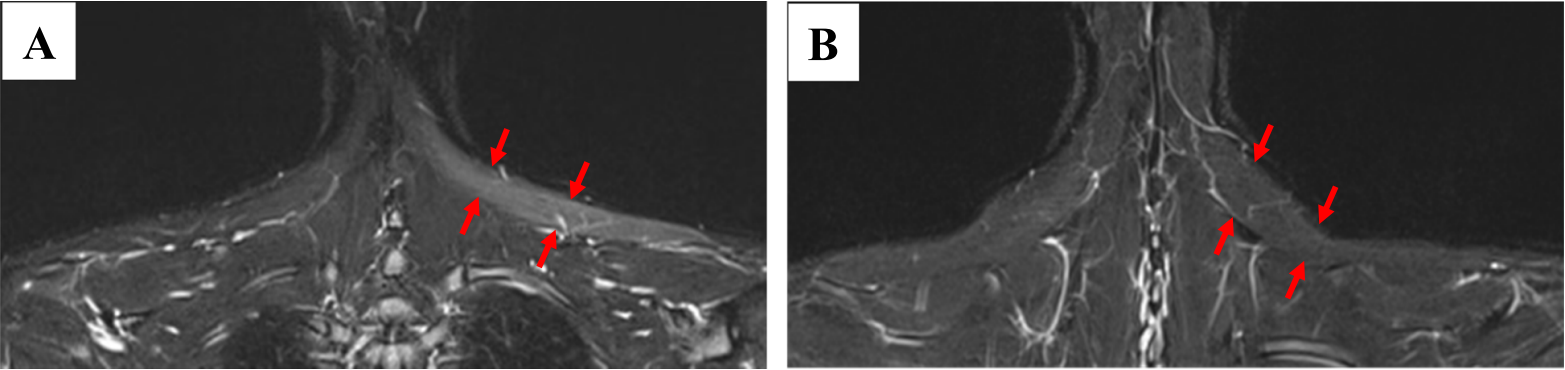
MRI findings. a A short T1 inversion recovery image showed high signal intensity in the left trapezius muscle before surgery (*red arrows*). b At 1 year postoperatively, the signal strength of the left trapezius muscle was reduced (*red arrows*)
